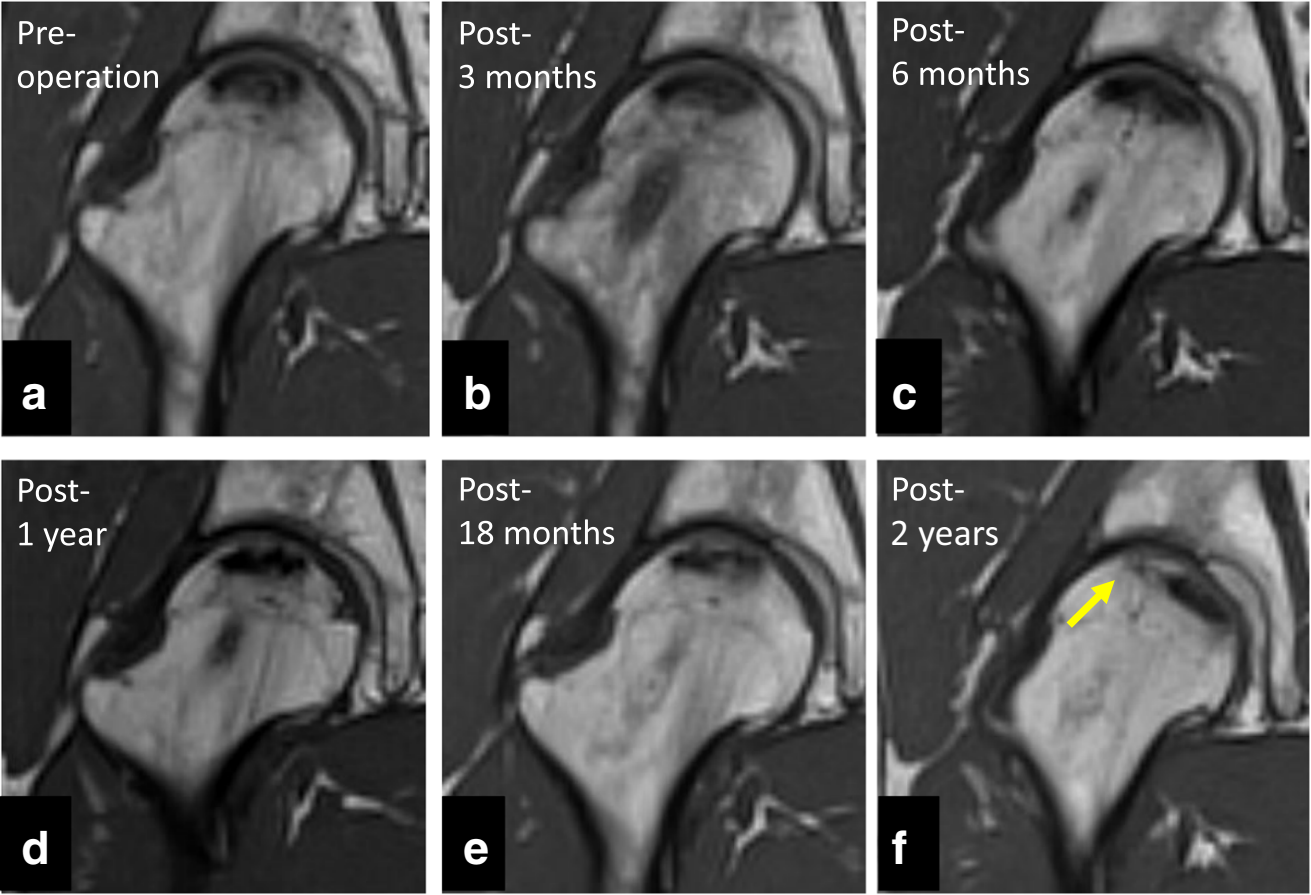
Representative magnetic resonance images. a Preoperative coronal T1-weighted magnetic resonance imaging (MRI) showing osteonecrosis of the femoral head (ONFH) that occupied the weight-bearing portion and extended laterally to the acetabular edge. b–d MRI scan of the ONFH area and the femoral neck region 6 months and 1 year postoperatively, showing continued low signal intensity, indicating the influence of the traumatic procedure. e MRI scan 18 months postoperatively, showing the first change of signal intensity at the drilling route. The drilling site at the femoral neck is changing to the normal signal intensity of the bone. f Most recent MRI scan 2 years postoperatively, showing almost normal signal intensity at the ONFH area. The area and size of ONFH decreased at the weight-bearing surface (*yellow arrow*)
